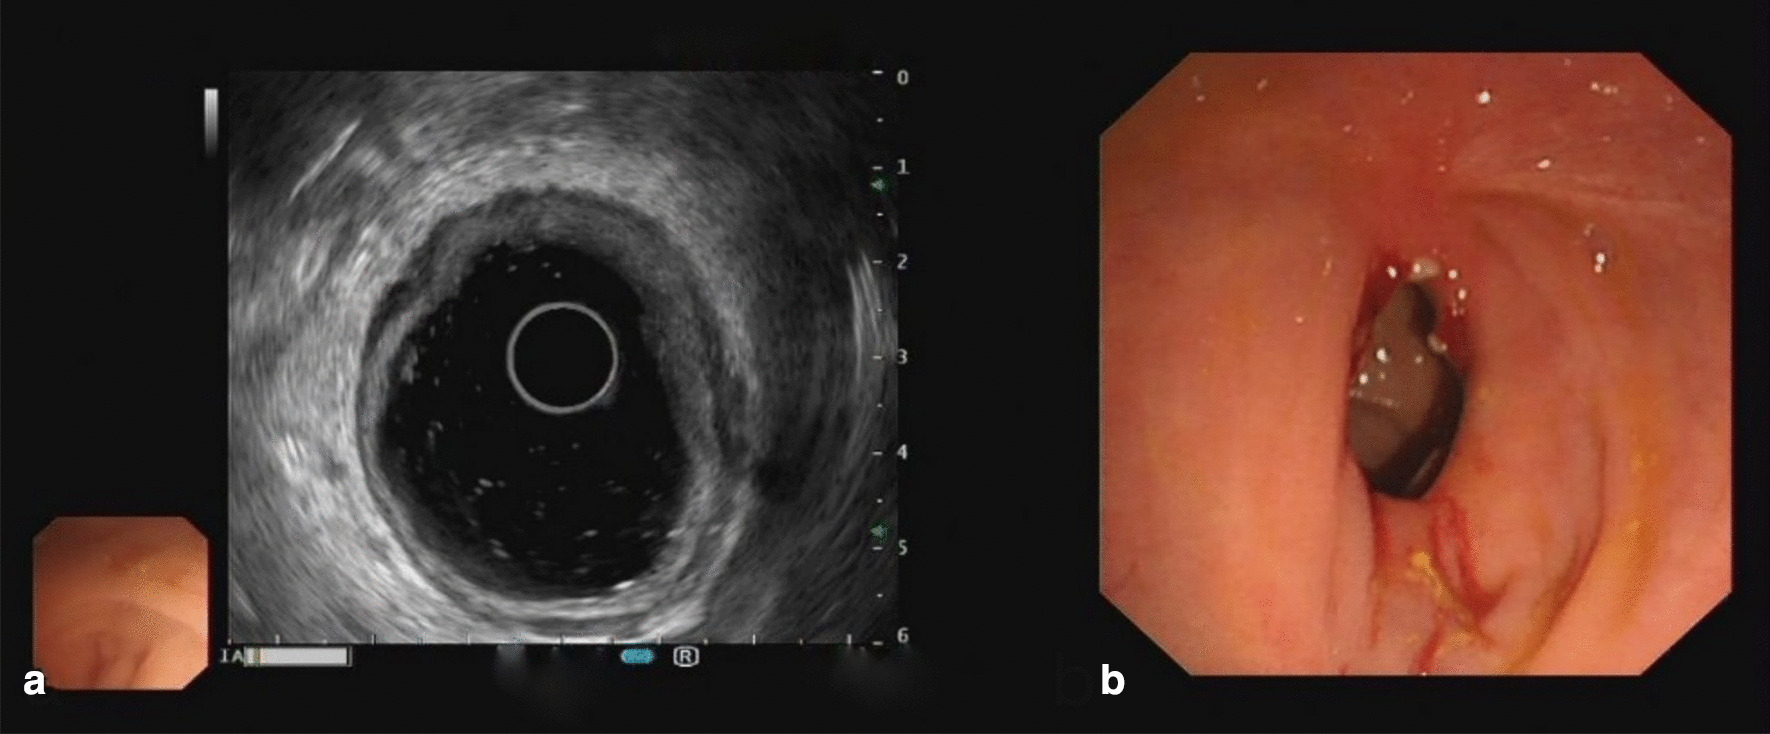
Endoscopic ultrasonography indicated a good prognosis. a The stratification of normal intestinal mucosal tissues at the original endoscopic recanalization site was visible under endoscopic ultrasonography. b The normal intestinal mucosa of the original endoscopic recanalization site was observed under endoscopic ultrasonography
